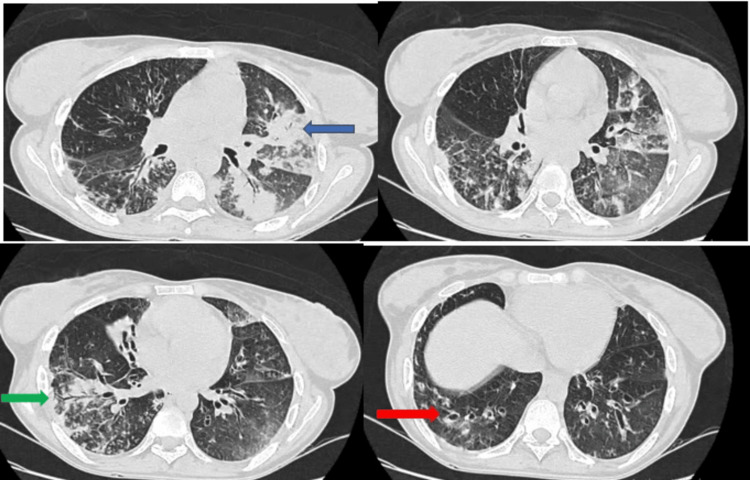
Axial-slice CT scans: foci of lung parenchymal condensation (blue arrow) surrounded by ground-glass areas, superimposed by peri-lobular reticulations associated with dilatations of cylidric (green arrow) and moniliform (red arrow) bronchi containing patchy mucoid impactions
